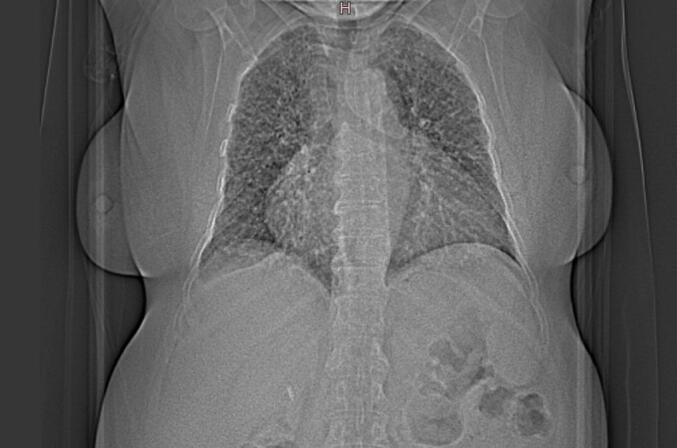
Chest radiography showing bilateral interstitial lung disease.
